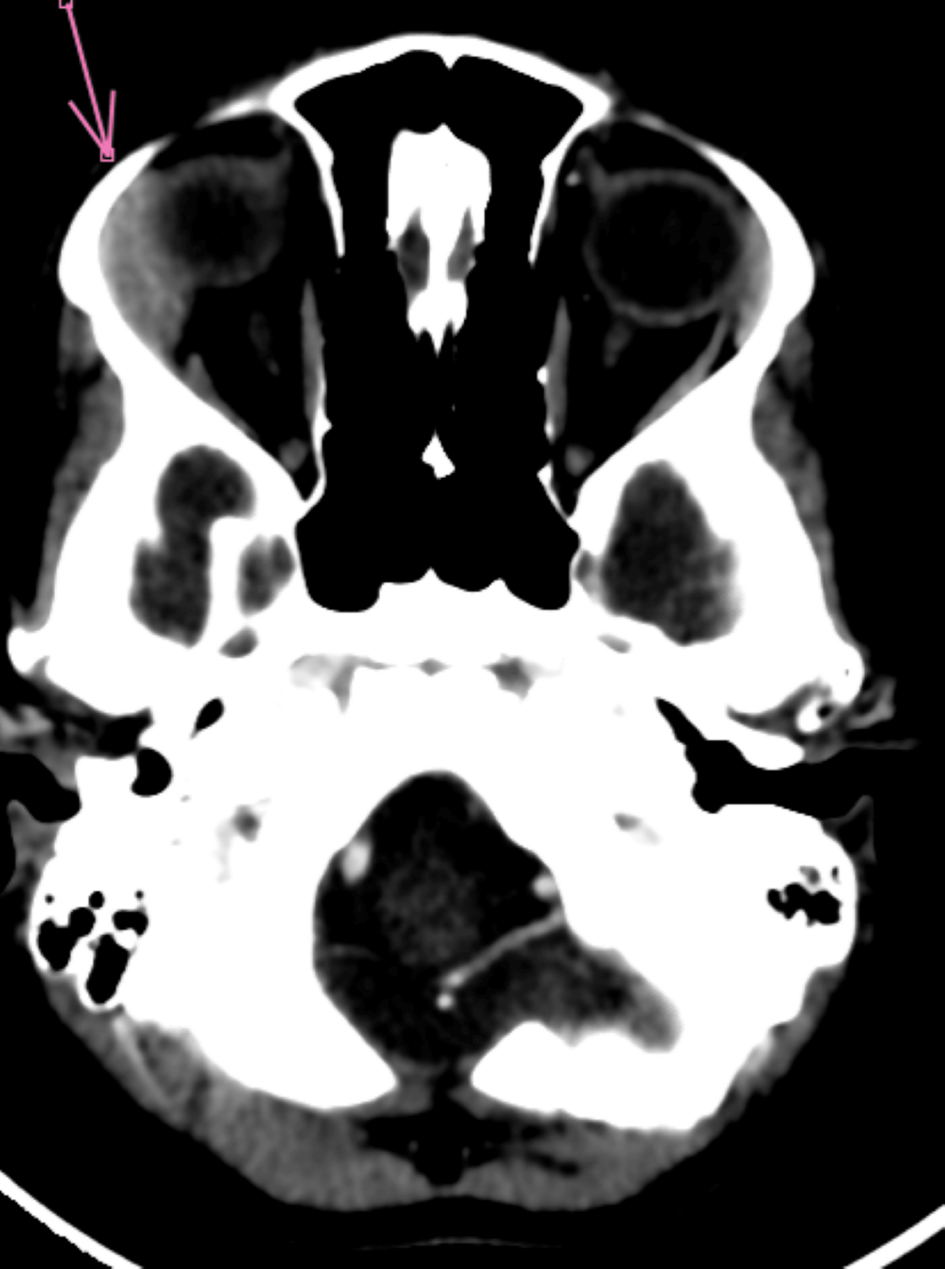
Axial contrast-enhanced brain-orbit CT scan showing infiltration of the lacrimal gland with extraconal extension
